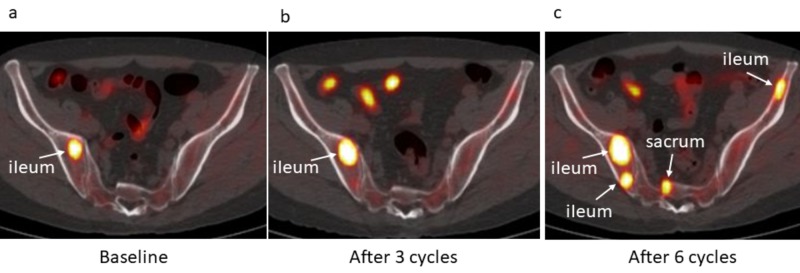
Selected axial 11C-choline PET/CT plane at (a) baseline, after (b) three and (c) six cycles. (a) A lesion in right ileum showed maximum standardized uptake value (SUVmax) of 8.1 on pretreatment ^11^C-choline PET/CT (arrow), reflecting bone metastasis. (b) The SUVmax of right iliac lesion increased to 14.3 on ^11^C-choline PET/CT after three cycles (arrow), reflecting the progression of bone metastasis. (c) The SUVmax of right iliac lesion kept high level (14.4) on ^11^C-choline PET/CT after six cycles. Several new uptakes appeared on the right ileum (SUVmax, 7.2), left ileum (SUVmax,5.0) and sacrum (SUVmax, 4.7), respectively (arrows), reflecting new bone metastases.
